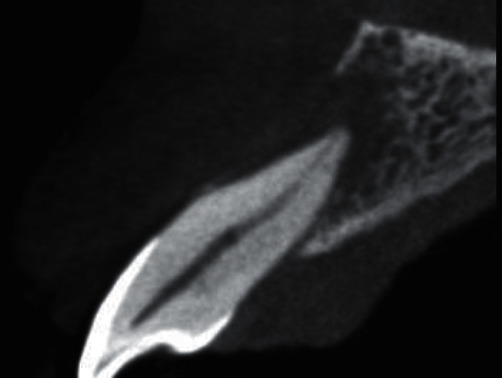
Preoperative CBCT showing extensive loss of bone on the labial aspect.
